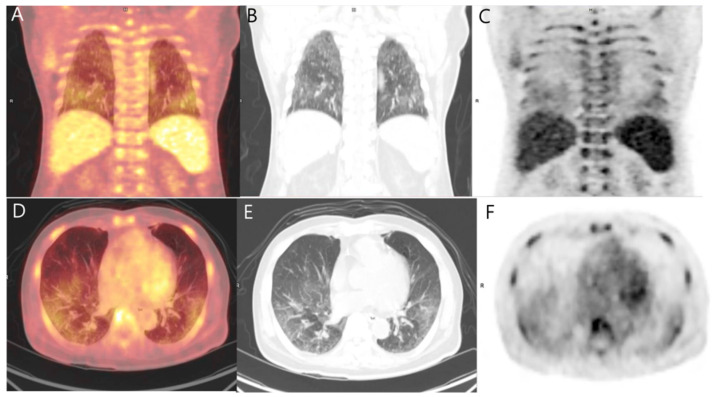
^18^F-FDG PET/CT coronal (A–C) and axial (D–F) images of the thorax demonstrated bilateral high-grade uptake in the posterior and inferior aspect of the lungs and diffuse low-grade uptake in the remainder of the lungs. Non-contrast CT image of PET/CT (B,E) revealed diffuse, hazy ground-glass opacities in the bilateral lower lungs.
